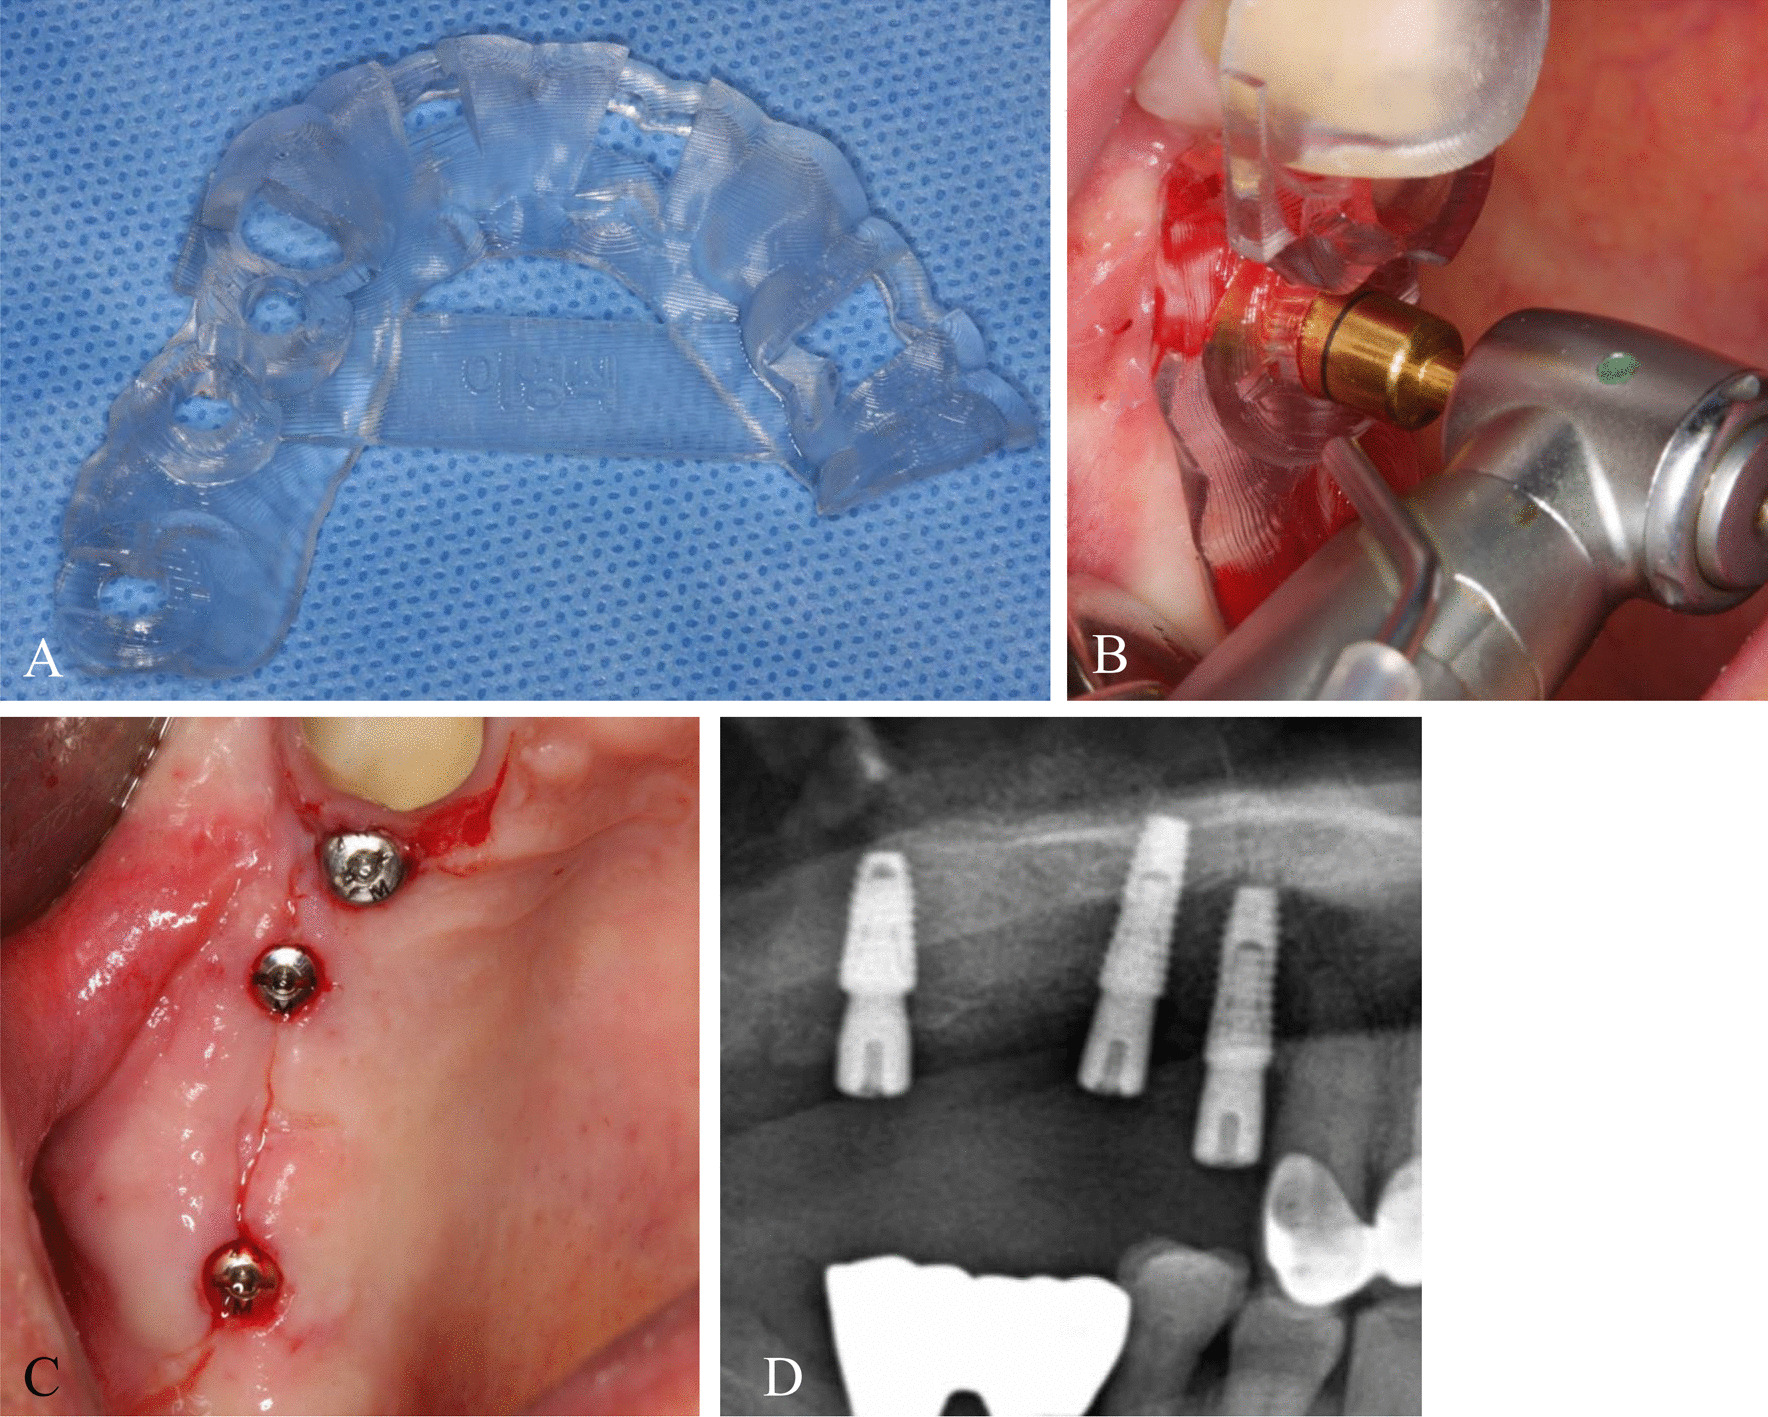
Guided implant placement with the surgical guide with the flapless approach. A Fabrication of the surgical guide for maxillary partial edentulism. B After the surgical guide was positioned in the surgical site, a 4.6-mm-diameter guided tissue punch was utilized for soft tissue removal with the indentation on the guide to stop drilling. C Following drilling by a 2.0-mm pilot-guided drill and a 4.2-mm tapered guided drill, the guided implant was placed in the planned location. D Postoperative radiography
